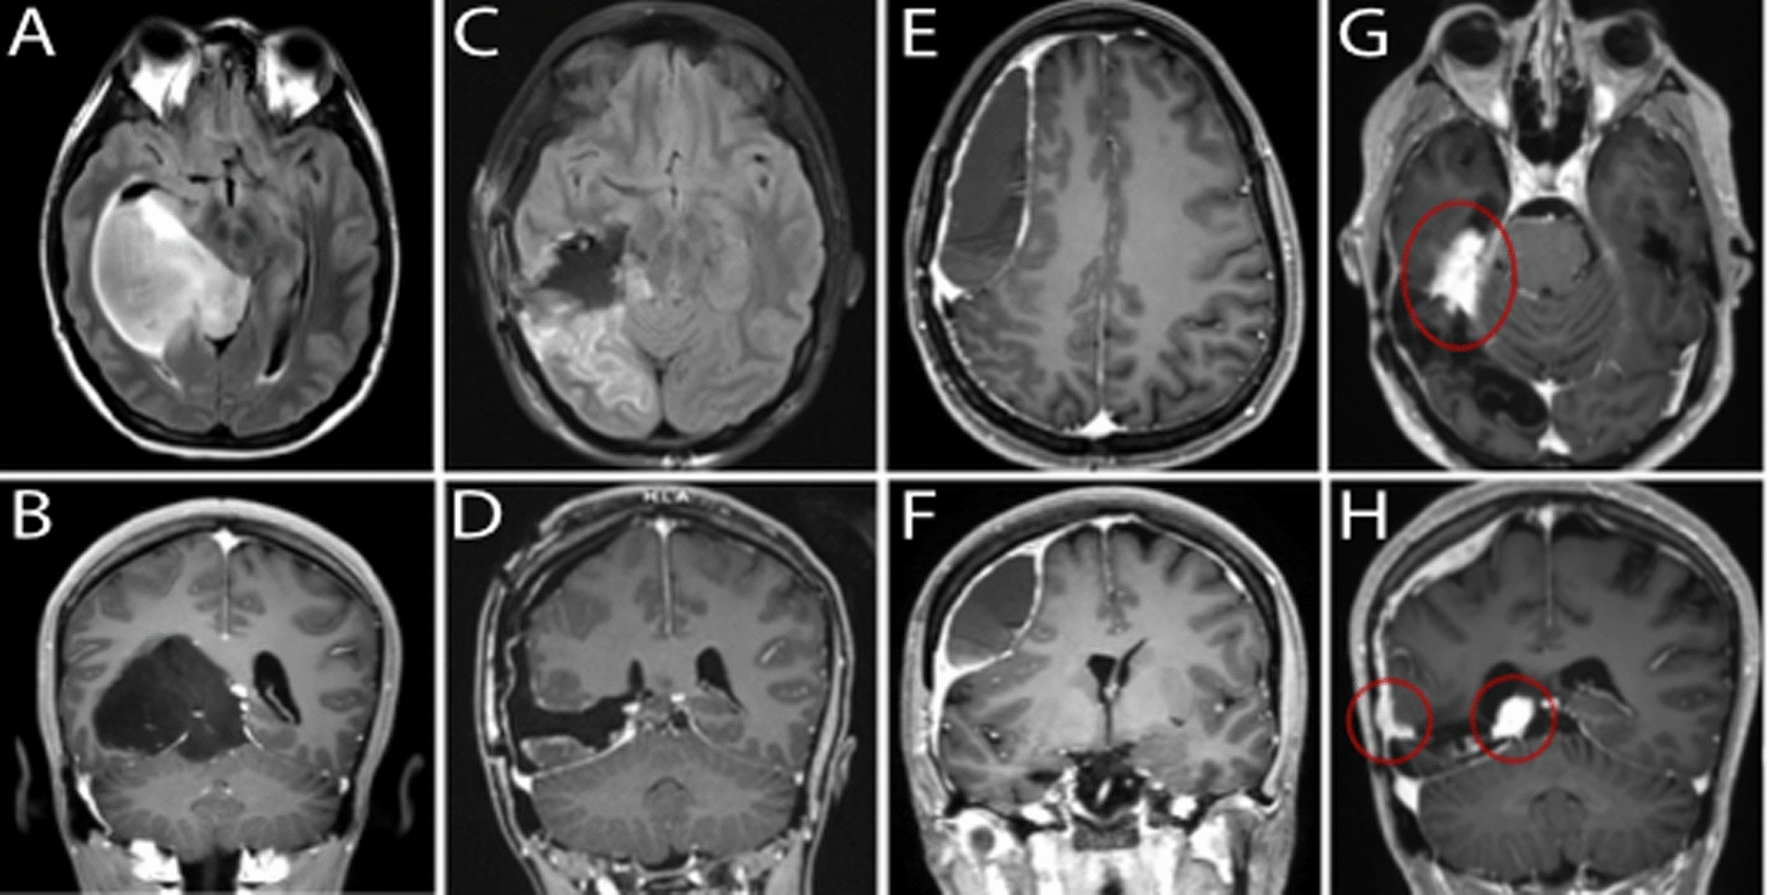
Magnetic resonance imaging over the course of treatment. Axial FLAIR (A) and coronal T1 post-contrast (B) images of tumor at time of presentation. Axial FLAIR (C) and T1 post-contrast (D) images following operative resection. Axial (E) and T1 post-contrast (F) images demonstrating subsequent formation of peripherally enhancing, hypodense extra-axial fluid collection with underlying mass effect and right to left midline shift. Axial (G) and coronal (H) T1 post-contrast images demonstrating multifocal recurrence including along the tentorium and convexity
